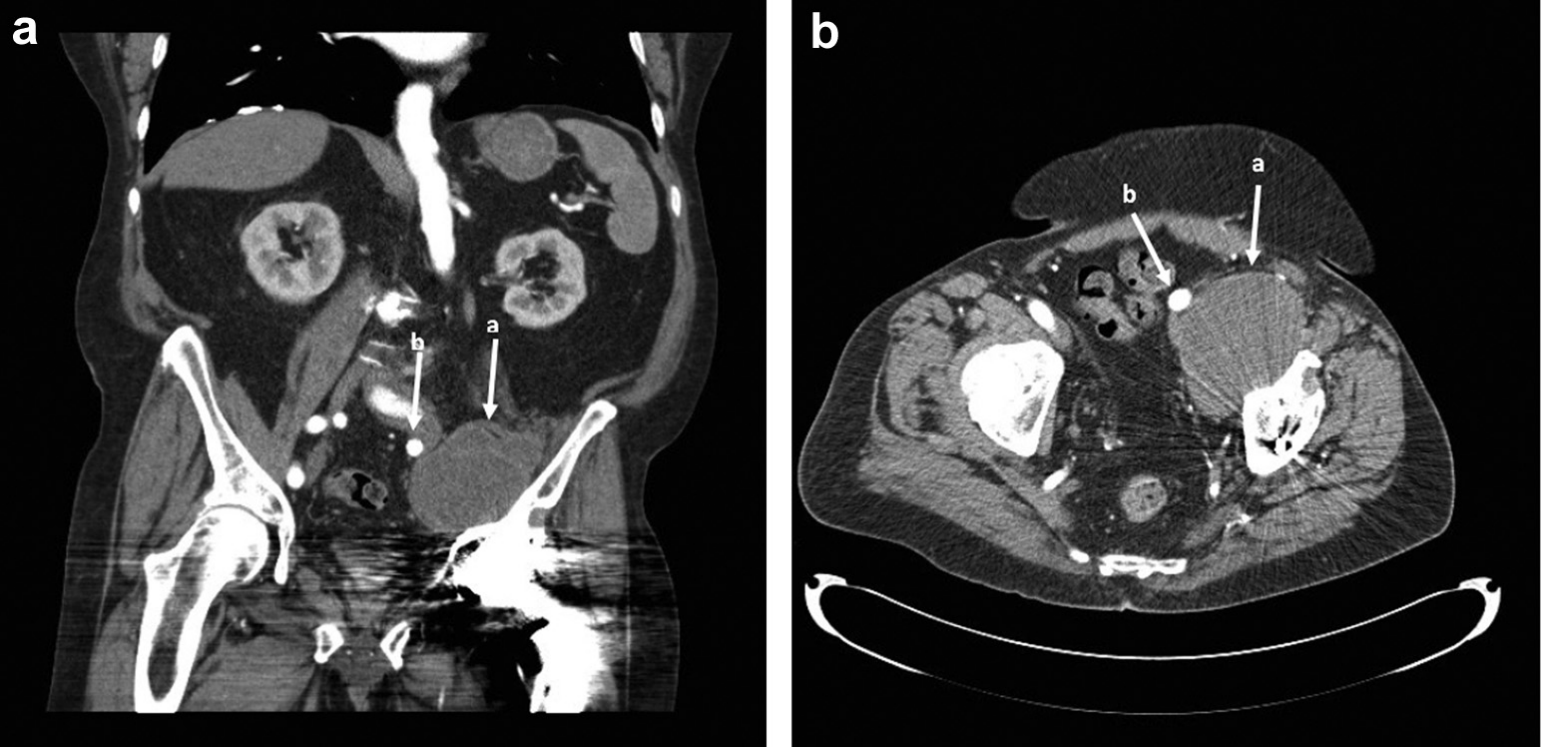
A contrast-enhanced angiographic computed tomography (CT) scan of the pelvis demarcating the left pelvis mass (a) in the iliac fossa that displaces the left common iliac artery (b) and vein medially. (a) Coronal view. (b) Axial view.
